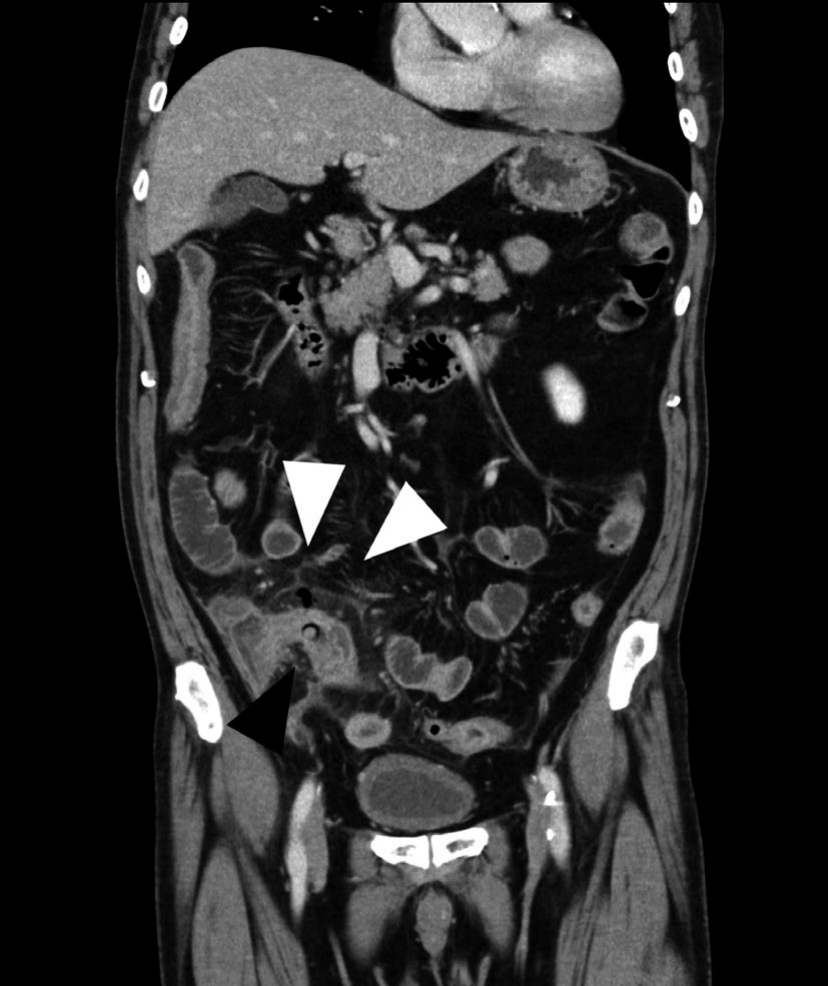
A 75‐year‐old man with type 2 diabetes mellitus and hypertension presented with a 5‐day history of worsened abdominal pain. His body temperature, blood pressure, pulse rate, and respiratory rate was 38.2°C, 146/80 mmHg, 92 beats/min, and 24 breaths/min, respectively. We also observed tenderness in the right lower abdomen, along with a muscular defense and attenuated bowel sounds. Laboratory tests revealed a white blood cell count of 8.71 × 10^9^/L (3.17–8.40) with 84.2% neutrophils and a high C‐reactive protein level of 161.5 mg/L (reference < 3.0). Contrast‐enhanced computed tomography revealed significant wall thickness in the terminal ileum, along with free air and dirty fat signs. A round, high‐density object surrounded by a low‐density area, which is a characteristic computed tomography finding for a tablet in a press‐through package (PTP), was observed in the terminal ileum (Figure 1). The patient was diagnosed with acute peritonitis and terminal ileum perforation caused by the PTP. Emergency laparotomy revealed a perforation in the terminal ileum and abscess formation in the thickened wall around the perforation. Subsequently, the patient underwent ileocecal resection, and the PTP was found in the resected ileum (Figure 2). The postoperative course was uneventful.
